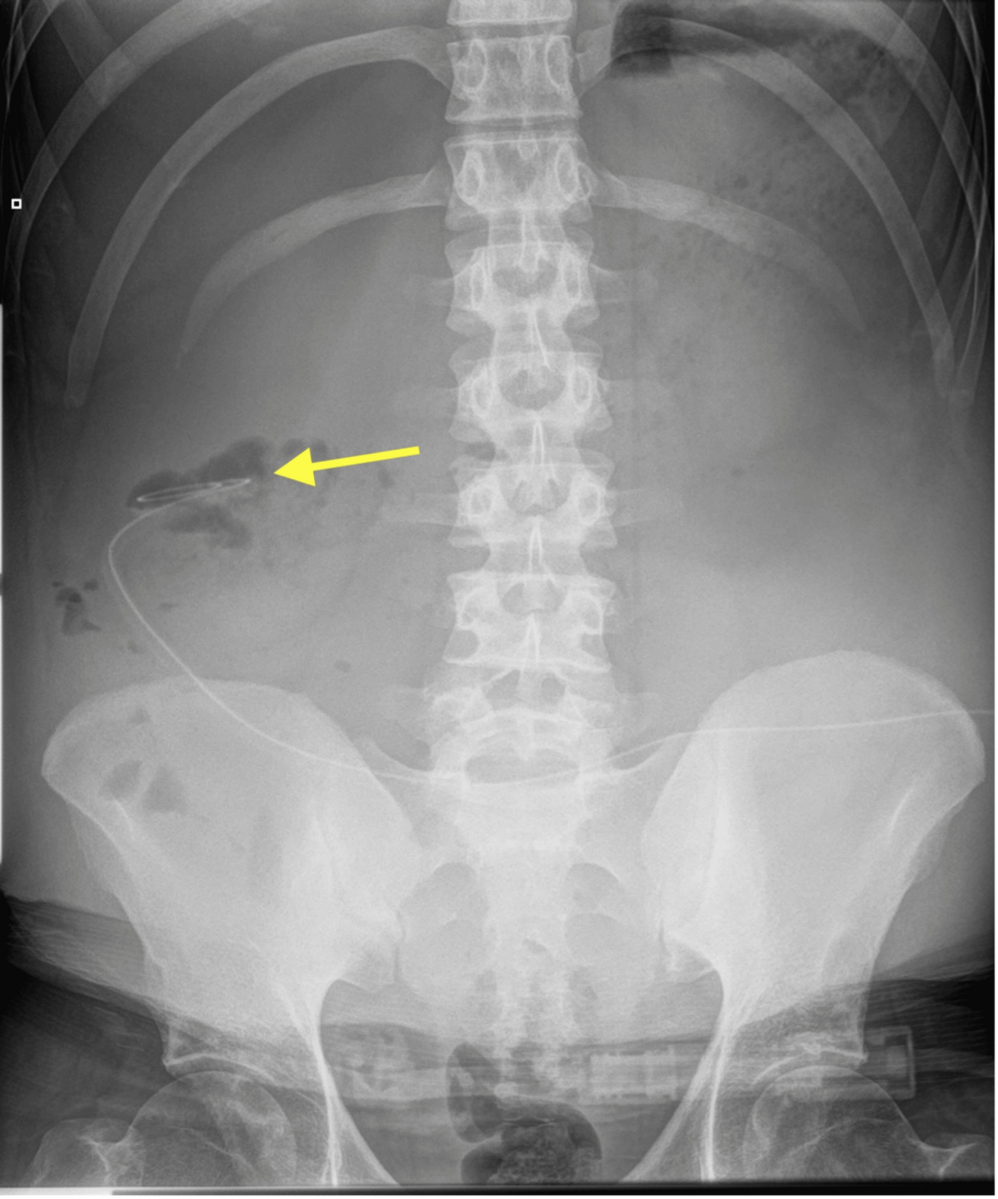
Abdominal X-ray showing the catheter tip in the right flank
